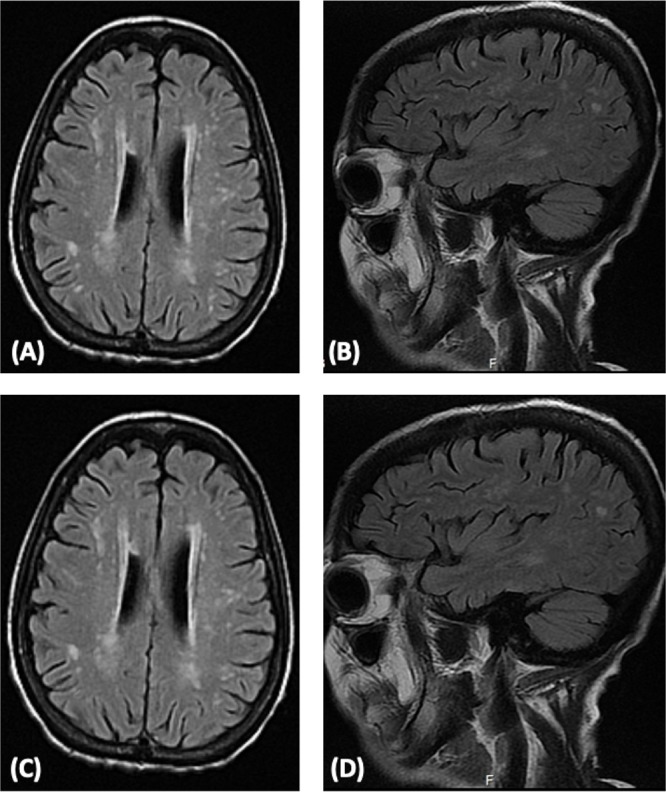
MRI Brain axial view (a) and sagittal view (b) T2 FLAIR suggest hyper-intense lesions in cortical, subcortical, periventricular and corpus callosum. MRI Brain axial view (c) and sagittal view (d) T2 FLAIR suggest no progression of the above mentioned hyper-intense lesions.
